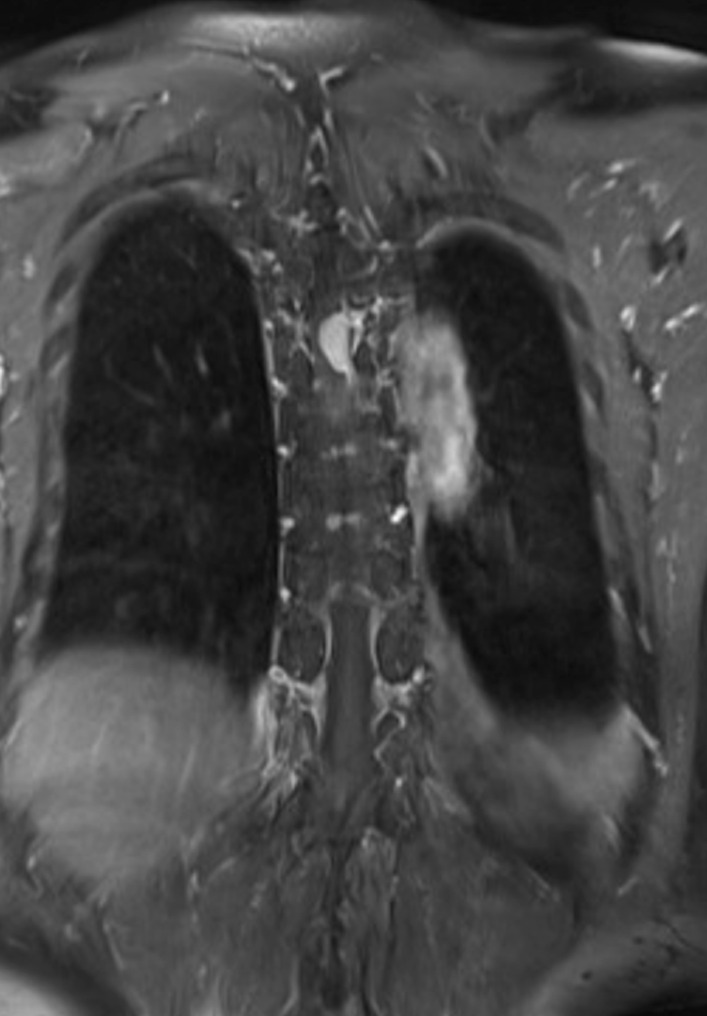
Coronal magnetic resonance image demonstrated a large oval-shaped mass at T6-7 level
